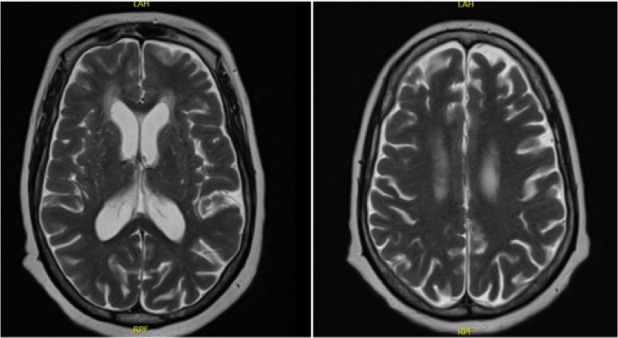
MRI from previous visit. Prominent pial enhancement throughout the brain, more in the right cerebral hemisphere with adjacent cortical FLAIR changes.
